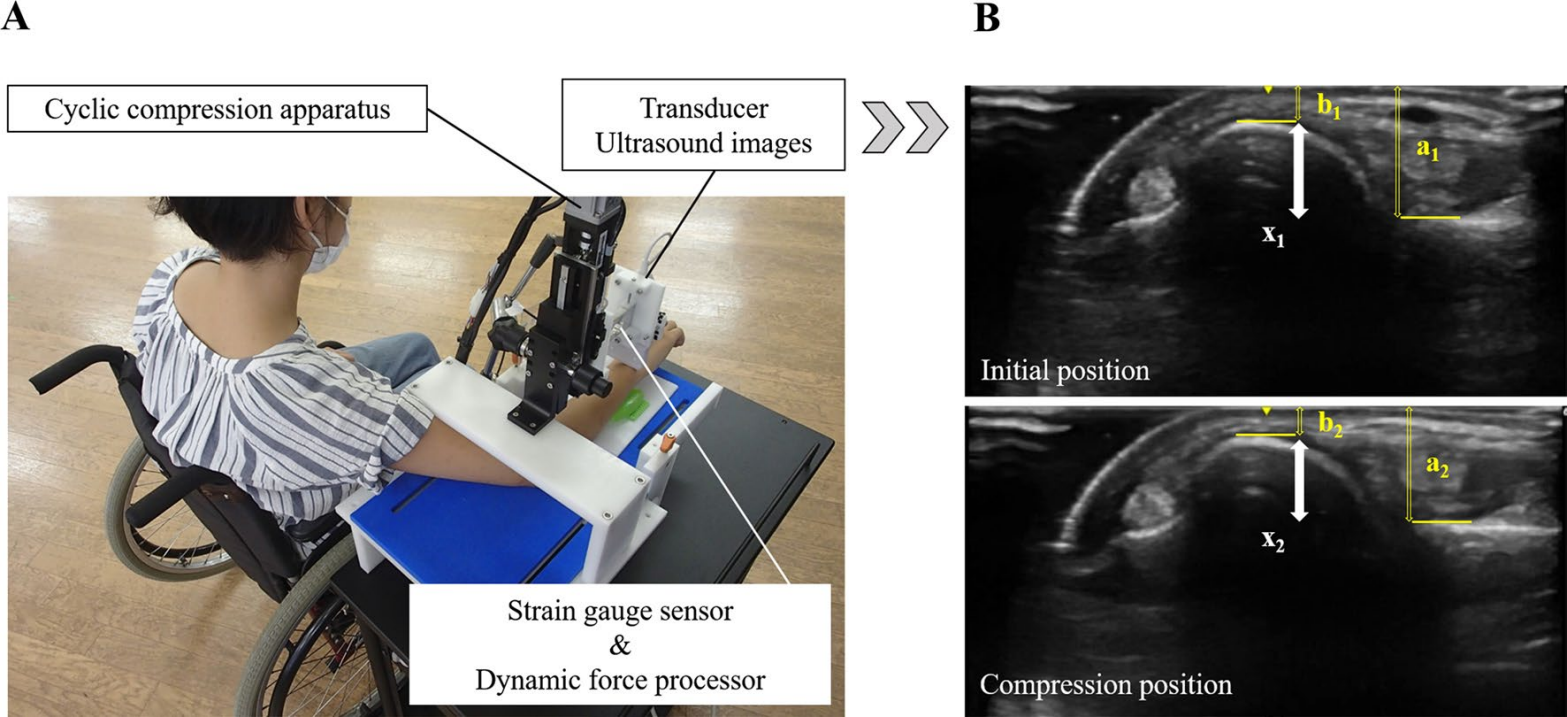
Evaluation of the distal radioulnar joint instability. A Force-monitor ultrasonography. B Sample of ultrasonographic images
